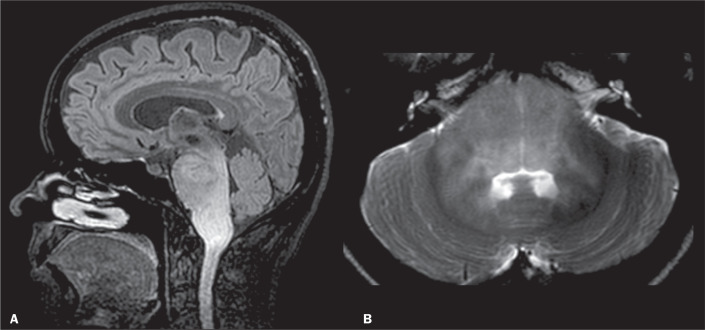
Diffuse midline H3K27M-mutated glioma. Sagittal FLAIR sequence
